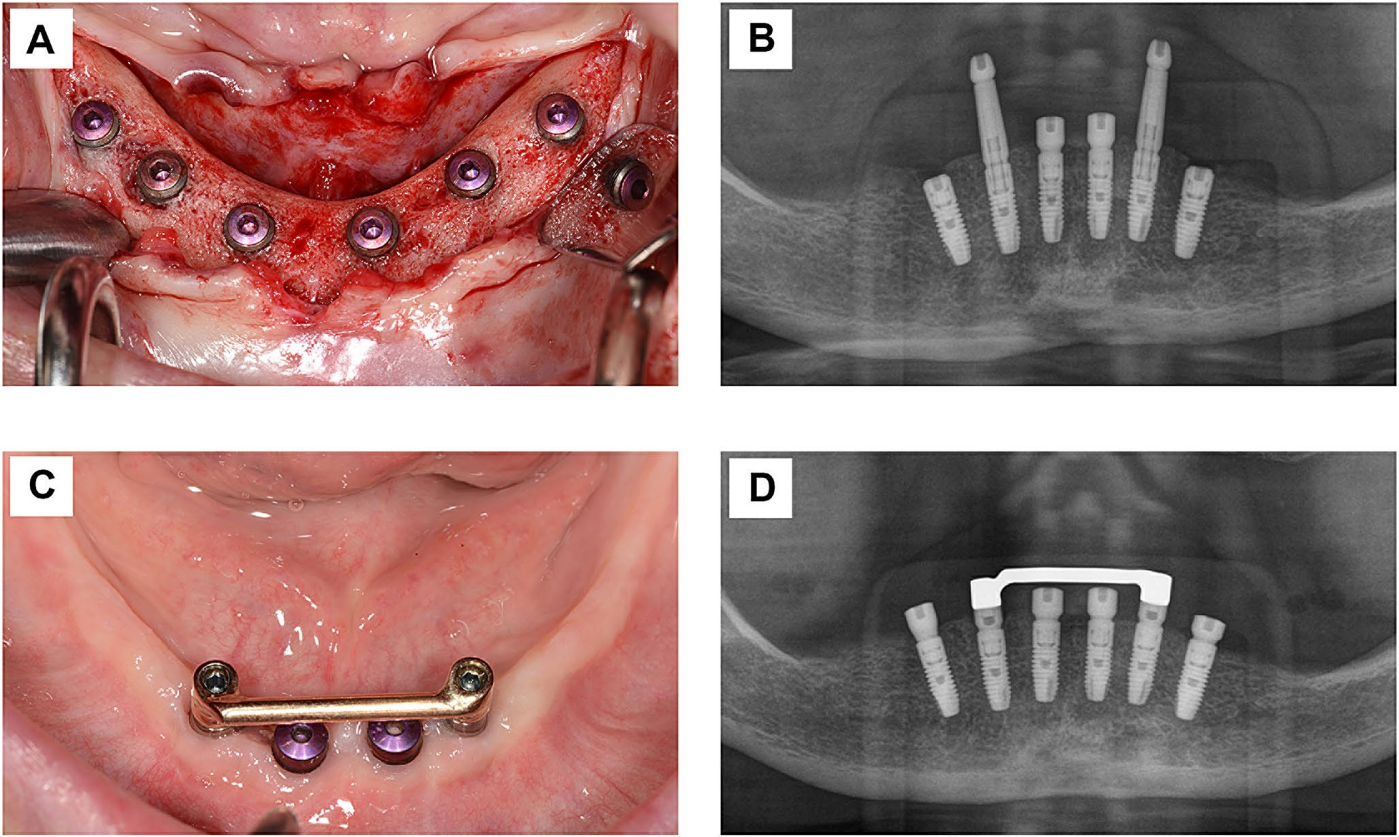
(A) Full-thickness flap after the re-entry procedure at Visit 8. (B) Radiograph showing implants with transfer abutments at positions 33 and 43, cover screws on distal implants, and healing abutments on mesial implants at Visit 2, following implant placement. (C) Clinical situation with integrated gold bar and healing abutments on transgingival implants at Visit 7. (D) Radiograph following re-entry surgery at Visit 8
